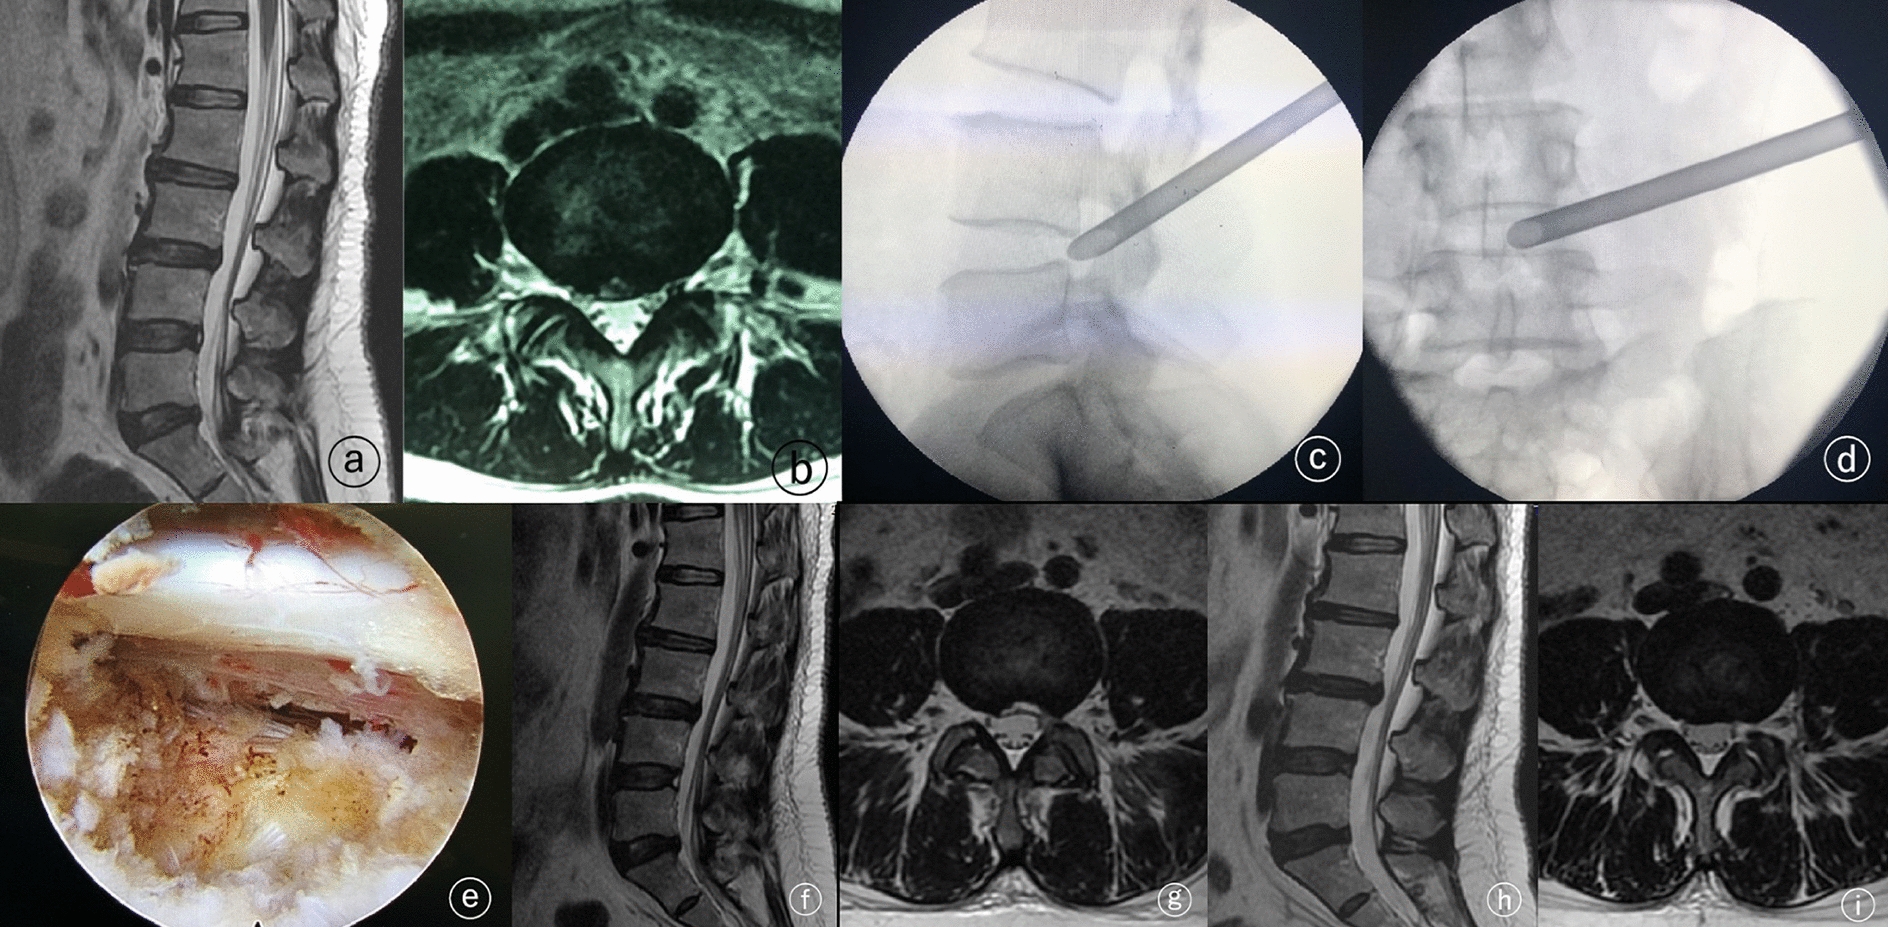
A, B High-intensity zone of L4/5 segment intervertebral disc annulus fibrosus can be seen in the preoperative lumbar MRI; C, D the working channel of spinal endoscopy during operation; E intraoperative images showed the “isolation zone” formed in the nerve root and ventral dura after endoscopic decompression; F, G one week after operation, the lumbar MRI was rechecked, and the signal of fibrillar ring tear disappeared; the range of ventral decompression of dura mater was sufficient; H, I the lumbar MRI was reexamined 10 months after operation, the tear of fibrous ring disappeared; the surgical scope of the "isolation zone" healed well
